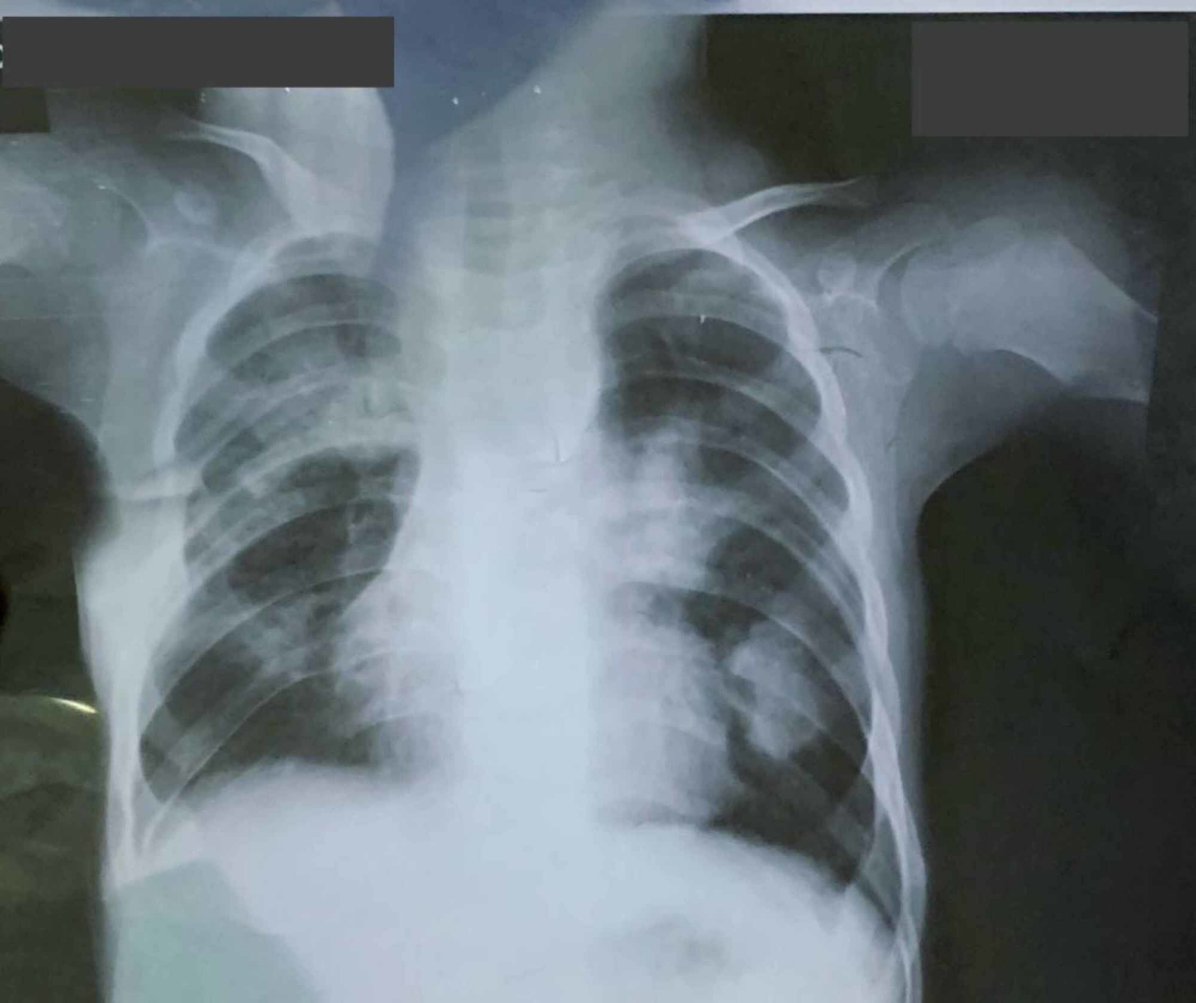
Chest X-ray of the patient before surgery
